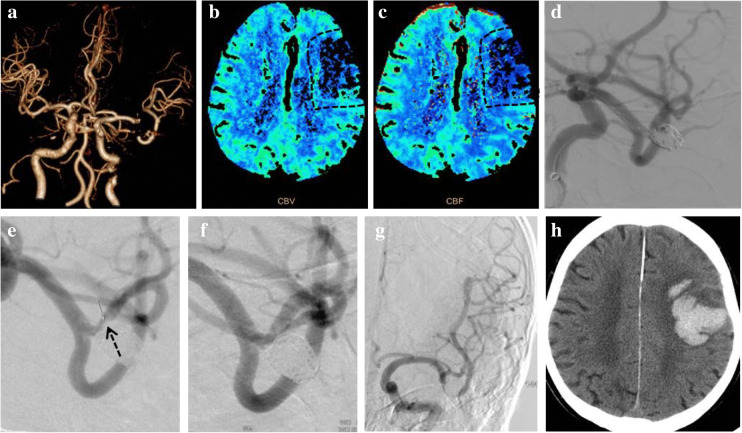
Images of case 2. a CT angiography: occlusion of the M1 upper branch of left MCA (white arrow). b CT perfusion: map of cerebral blood volume revealing low volume in the left frontal and parietal lobe (white dotted frame). c CT perfusion: map of cerebral blood flow revealing decreased flow in the left frontal and parietal lobe (white dotted frame). d Cerebral angiography: partial recanalization of the M1 upper branch with recovering forward flow and coil embolization assisted with stent for unruptured bifurcation aneurysm. e Cerebral angiography: residual thrombus in M1 bifurcation (black arrow). f Cerebral angiography: thrombus disappeared completely. g Cerebral angiography: complete recanalization of the upper branch. h Follow-up non-contrast CT at 24 h after IVT: left frontal and parietal hematoma
